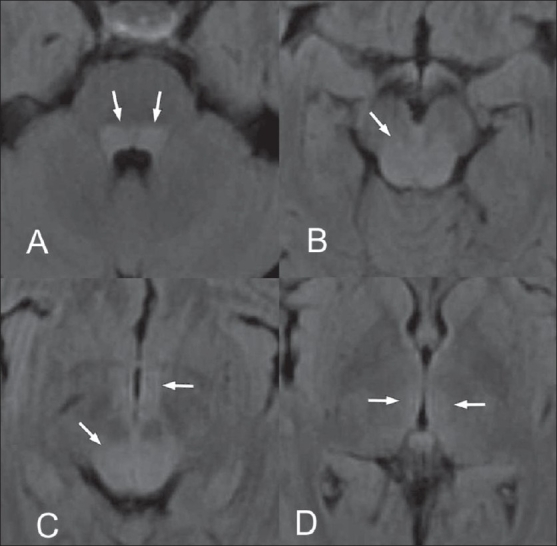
Axial FLAIR MRI images of the brainstem and thalamus reveal hyperintense lesions (arrows) involving the pontine tegmentum (A), most of the midbrain (B, C), hypothalamus (C) and medial parts of the thalami (D)
